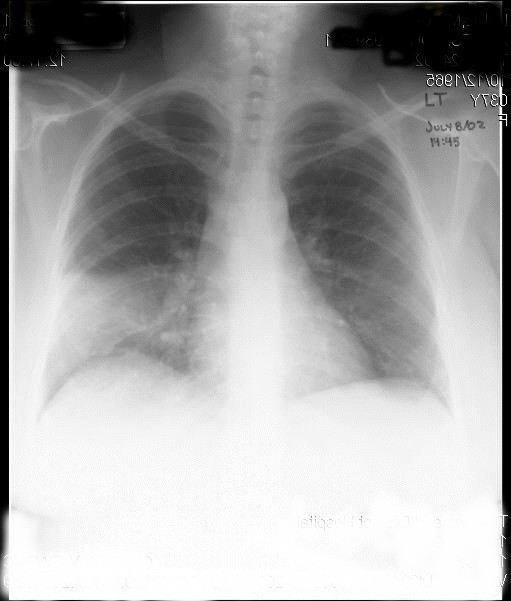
Chest X-ray (PA view) at initial presentation showing early right lower lobe consolidation.
